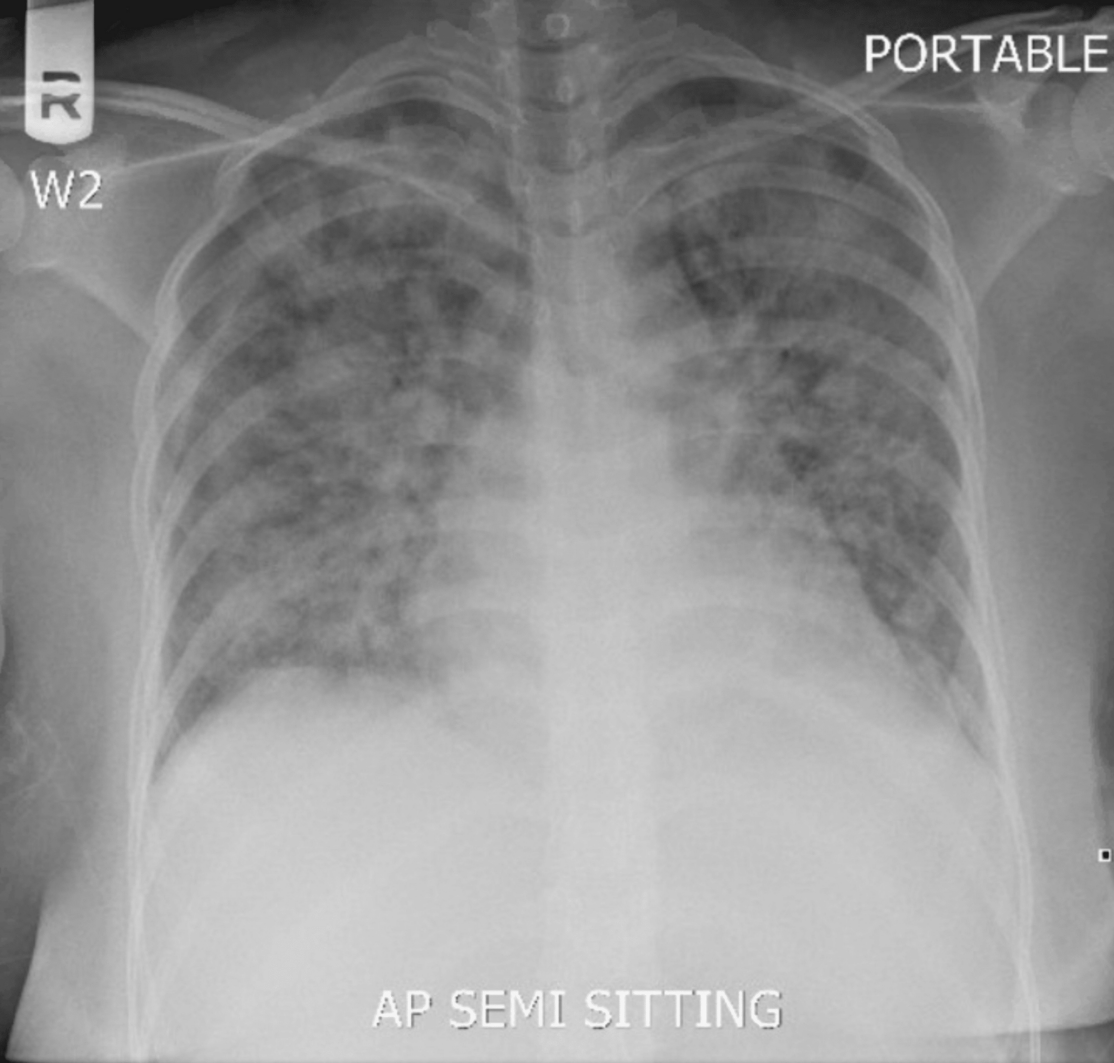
Chest X-ray showing a significant increase in the widespread areas of airspace opacities in the bilateral lung zones, as compared to the previous chest X-ray
